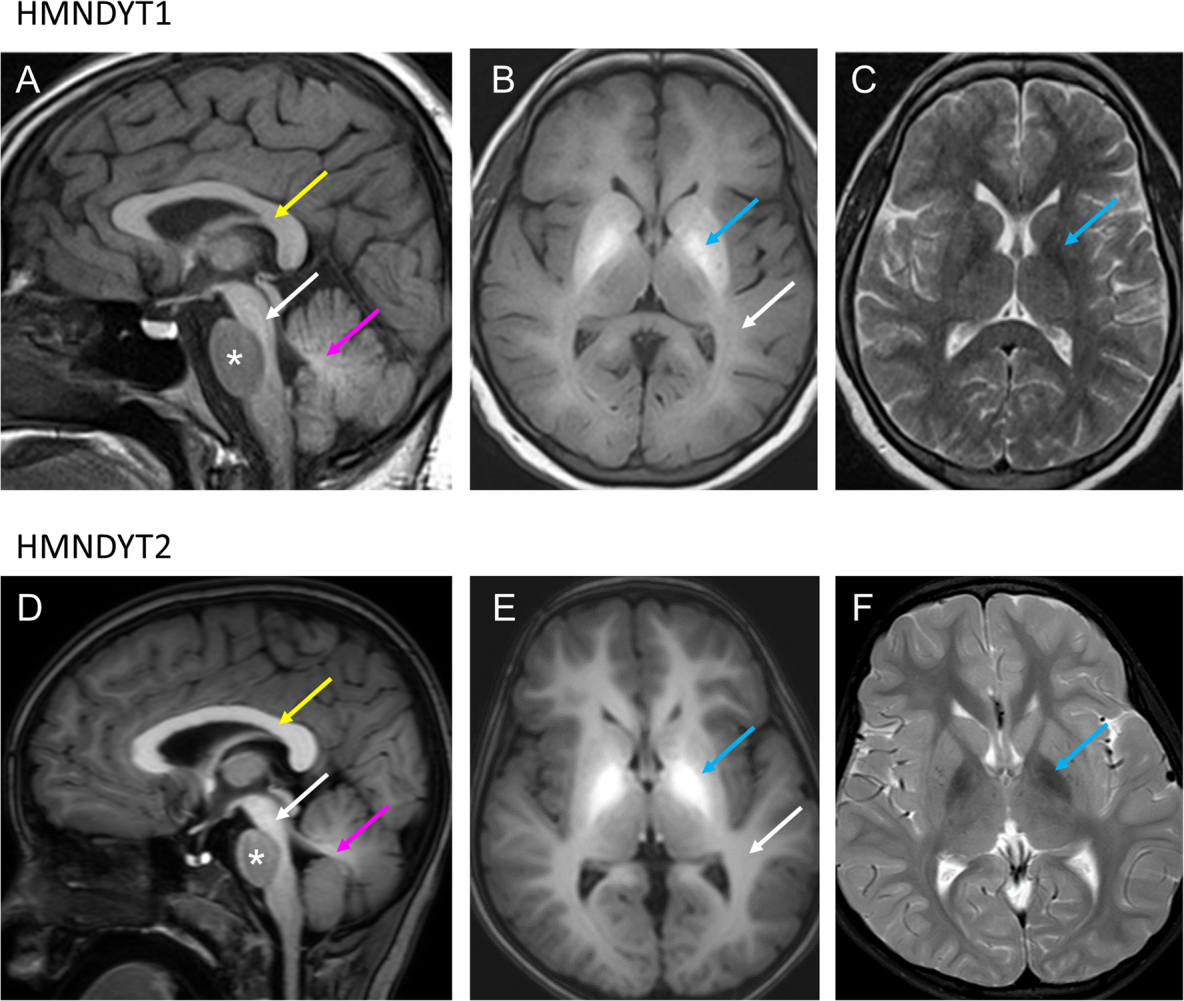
Characteristic appearances of Mn deposition in HMNDYT1 and HMNDYT2 on brain MRI. a–c Individual with HMNDYT1. d–f. Individual with HMNDYT2. a, d T1-weighted sagittal imaging showing hyperintensity of the white matter of the corpus callosum (yellow arrow), cerebellum (pink arrow) and the dorsal pons (white arrow) with characteristic sparing of the ventral pons (*). b, e T1-weighted transverse imaging showing hyperintensity of the globus pallidus (blue arrow) and cerebral white matter (white arrow) bilaterally. c, f T2-weighted transverse imaging showing hypointensity of the globus pallidus (blue arrow) bilaterally corresponding to T1-hyperintensities [22••, 26••]. (Reprinted from: Tuschl, K. *et al*. Syndrome of Hepatic Cirrhosis, Dystonia, Polycythemia, and Hypermanganesemia Caused by Mutations in SLC30A10, a Manganese Transporter in Man, Am. J. Hum. Genet, 90 (2012) 457–466; with permission from Elsevier) [22••]. (Reproduced from Tuschl, K. *et al*. Mutations in SLC39A14 Disrupt Manganese Homeostasis and Cause Childhood-Onset Parkinsonism-Dystonia, Nat Commun, 7: 11601 doi: 10.1038/ncomms11601 (2016); Creative Commons user license http://creativecommons.org/licenses/by/4.0/) [26••]
